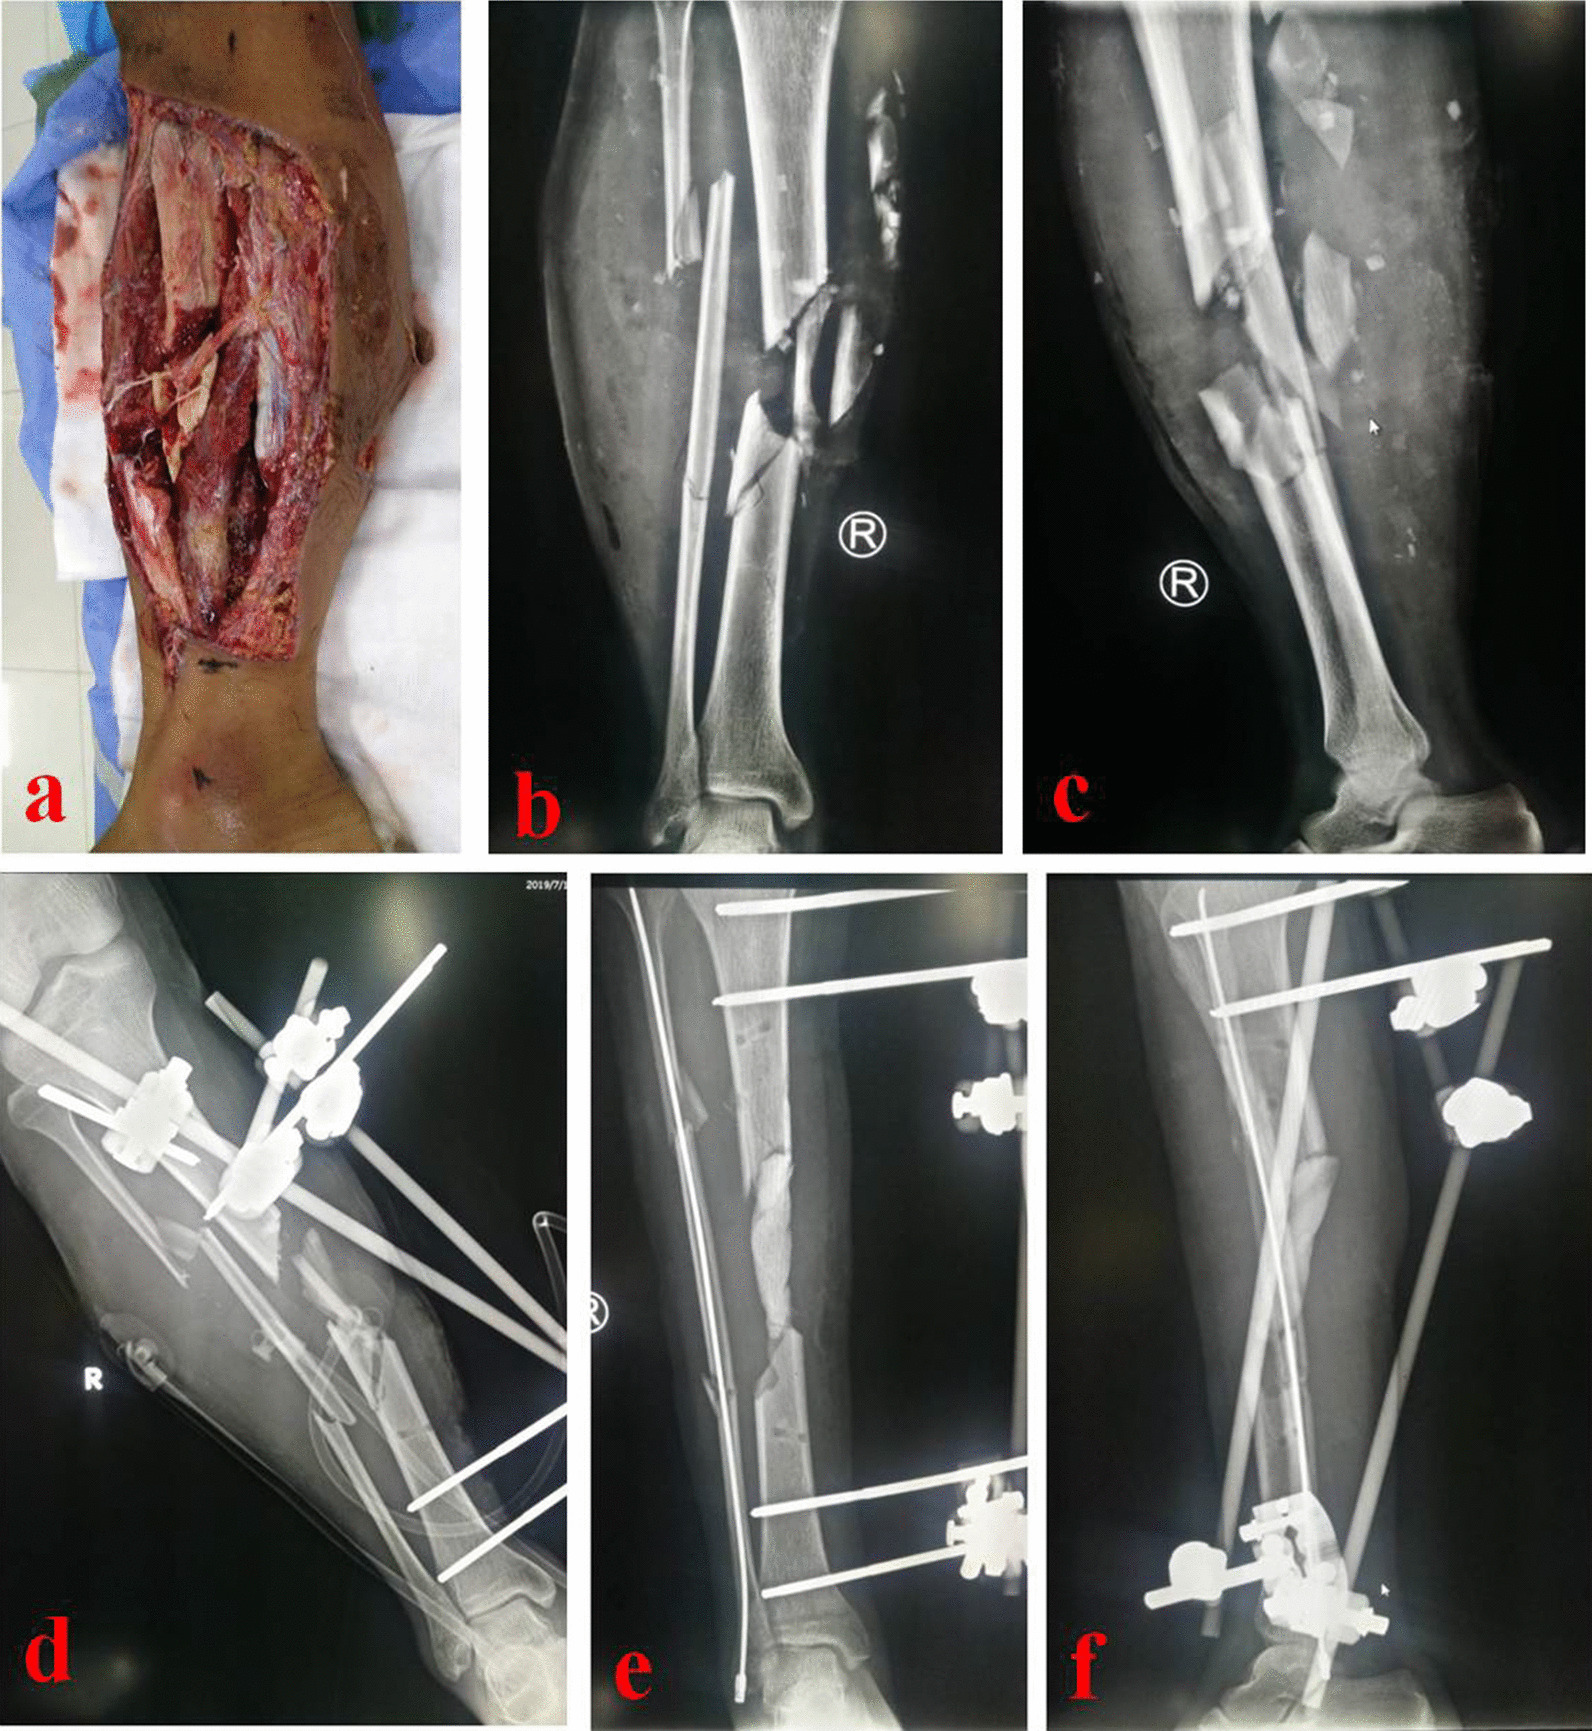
A 35-year-old male suffered from a car accident and was diagnosed as tibial open fracture. a Appearance before debridement, b and c X-rays of the affected tibia before debridement; d–f after radical debridement and soft tissue restoration, an 11 cm of bone defects was left
